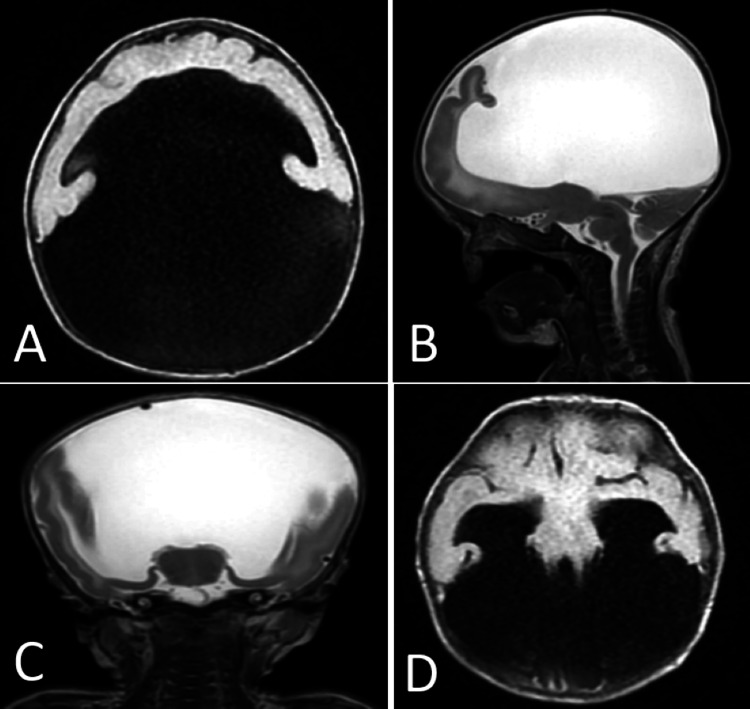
Brain MRI in axial T1 (A) and sagittal T2 (B) views shows residual brain parenchyma limited to an anterior-inferior lamina associated with a single ventricle communicating with a large dorsal cyst. Coronal T2 (C) and axial T1 (D) images show the absence of an interhemispheric fissure and non-separated central gray nuclei and thalami at the midline
